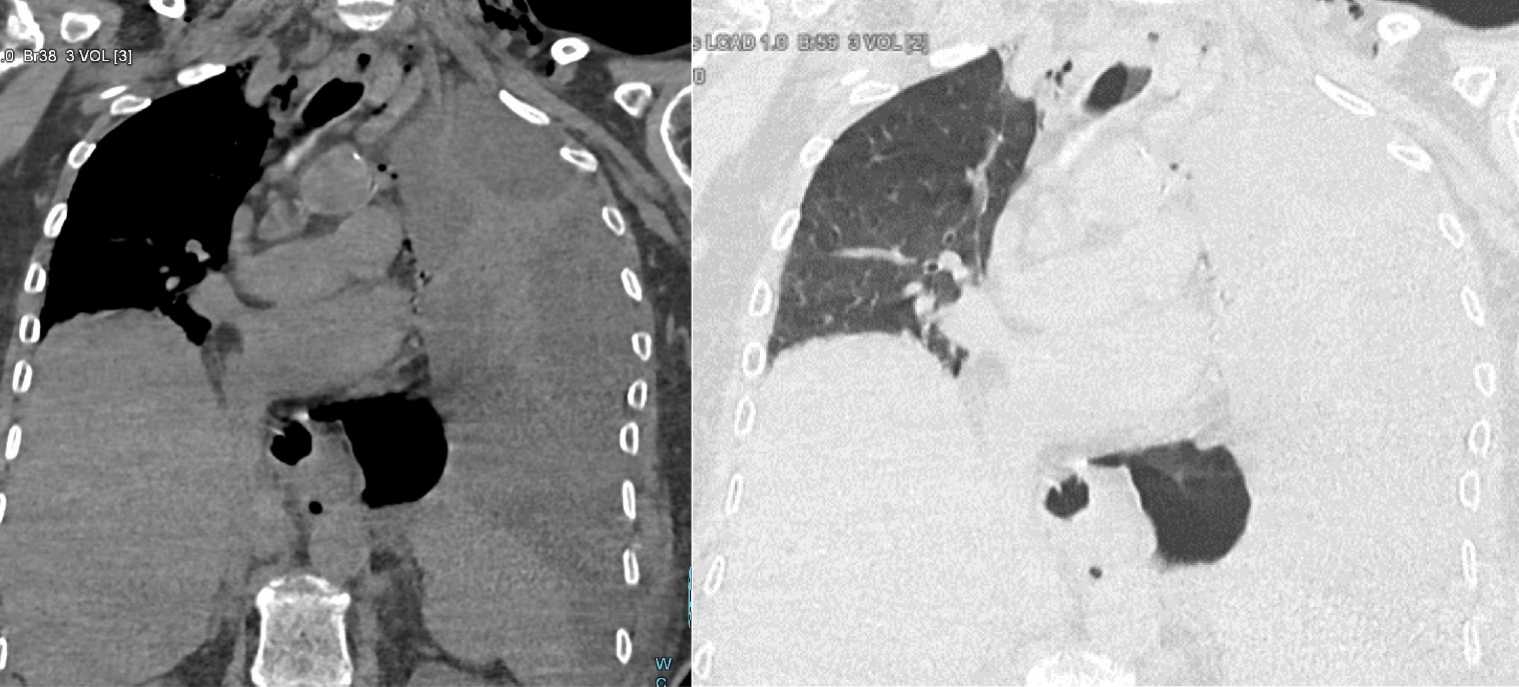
Follow‑up chest CT. Coronal reformatting in the mediastinal and pulmonary windows showing encapsulated pleural effusion (empyema) and complete left lung atelectasis. Source: Charleroi University Hospital.
